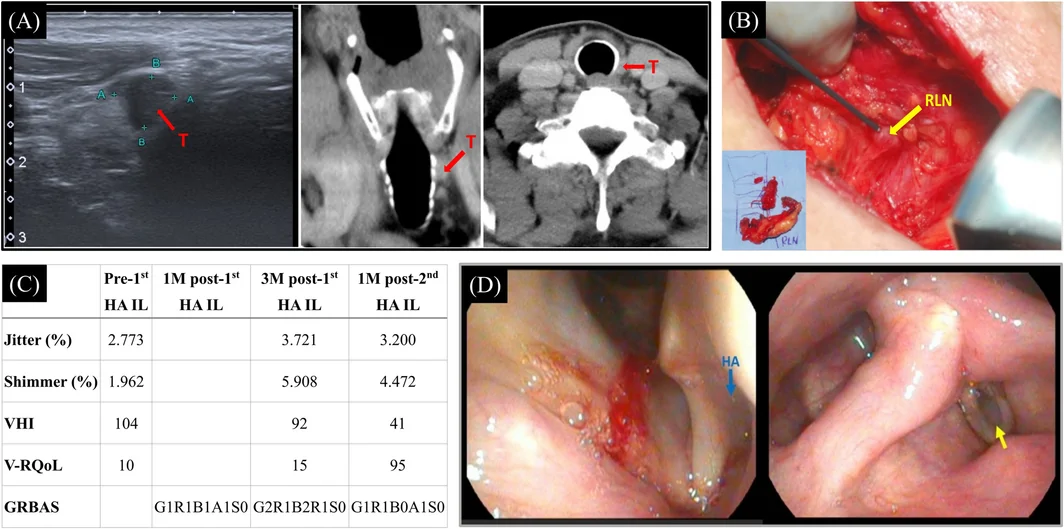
(A) Preoperative image—A heterogenous thyroid tumor (T) was noted on neck ultrasonography and computed tomography. Tumor invasion to the left tracheoesophageal groove was demonstrated. (B) Intraoperative findings—the left recurrent laryngeal nerve (RLN) was invaded by the tumor. (C) Objective and subjective voice outcomes of each hyaluronic acid (HA) injection laryngoplasty (IL), including jitter, shimmer, voice handicap index (VHI), voice‐related quality of life (V‐RQOL), and GRBAS scores. (D) Pre‐supplemental laryngofiberscope—performed at 3 months after the first injection still showed HA (blue arrow), but a glottic gap (yellow arrow) was noted when phonation. Therefore, a supplemental HA IL is then performed.
